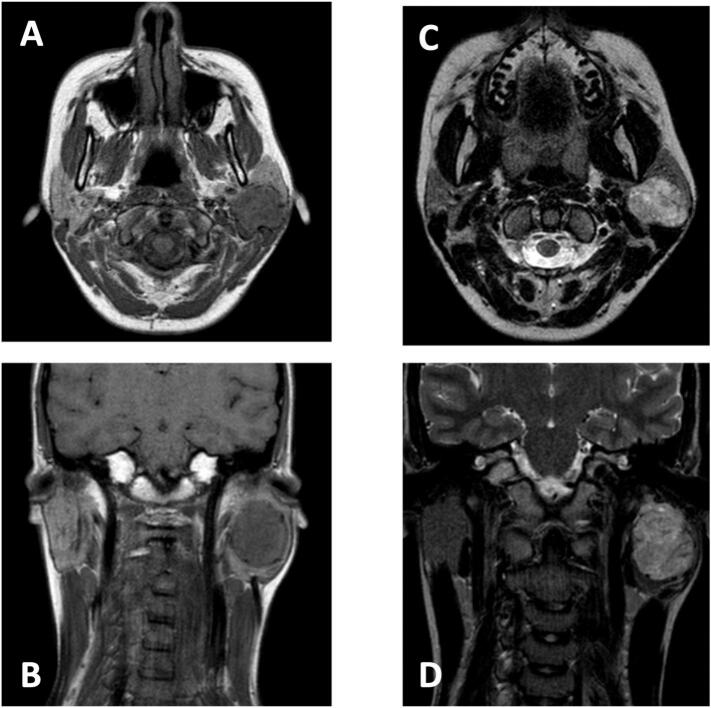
Magnetic Resonance Imaging showed an oval-shaped neoformation, medium-low intensity at T1 on axial (A) and coronal (B) scans, high intensity at T2 on axial (C) and coronal (D) scans.
